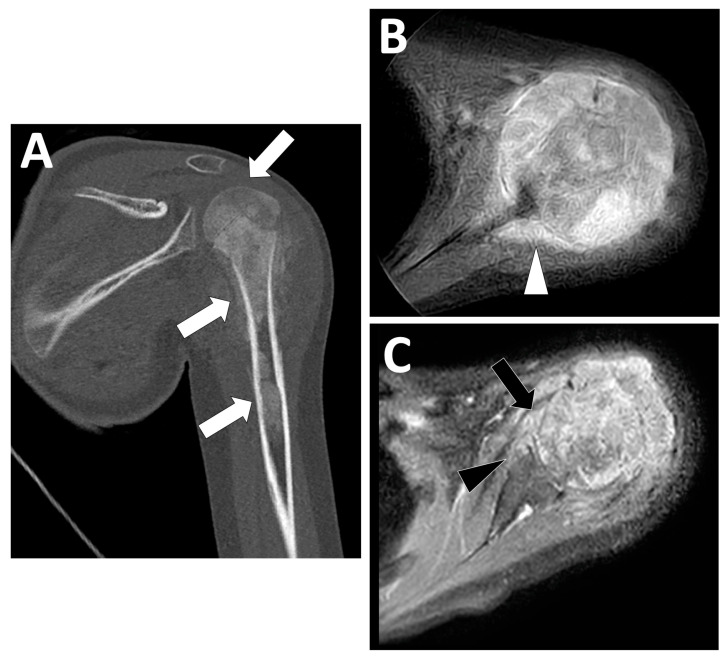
Transphyseal and intra-articular spreading observed during the initial staging of a high-grade osteoblastic osteosarcoma of the proximal left humeral bone diagnosed in a 12-year-old girl. (A) Coronal view of the initial CT scan showing an extensive tumor (>15 cm long) involving the epiphysis, metaphysis, and diaphysis of the humerus (white arrows). (B,C) Axial contrast-enhanced T1-weighted imaging showing marked contrast enhancement surrounding the scapula (white arrowhead) with periosteal enhancement, fulfilling of the articular recessus (black arrow) and erosions of the anterior side of the glenoid (black arrowhead).
